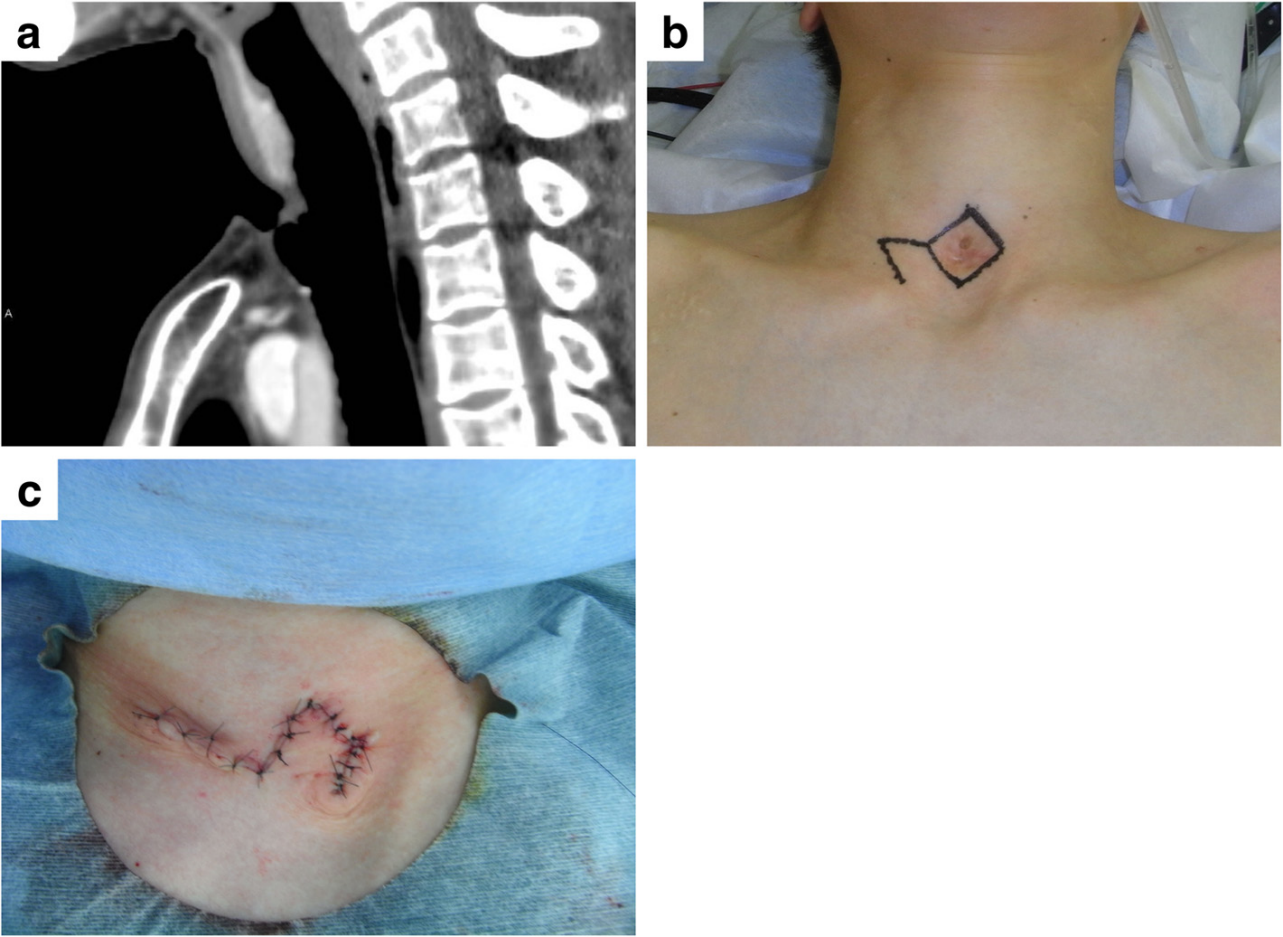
Preoperative and operative findings. a Cervical sagittal computed tomography showed a tracheocutaneous fistula. b The skin incision was designed as a box around the fistula and two sequential straight lines. c A single flap was elevated and rotated to cover the skin defect, and the skin was sutured layer by layer
